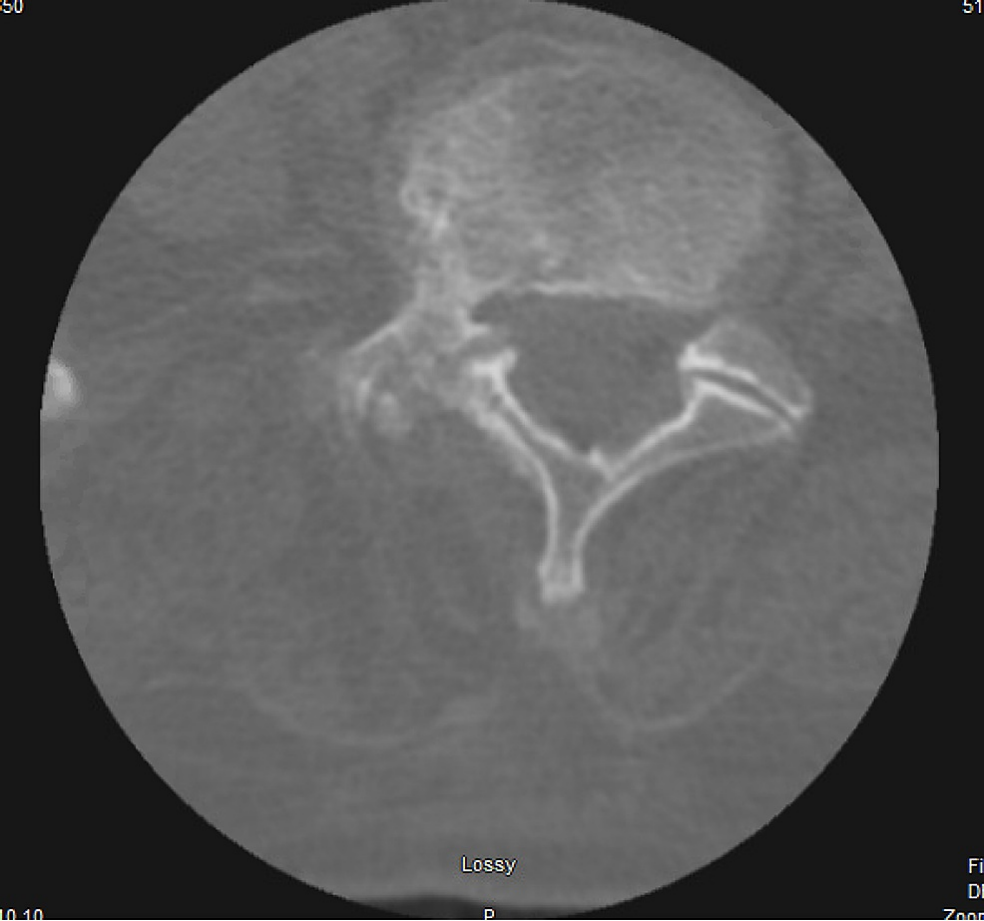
CT scan without contrast showed moderate disc bulging CT, computed tomography
